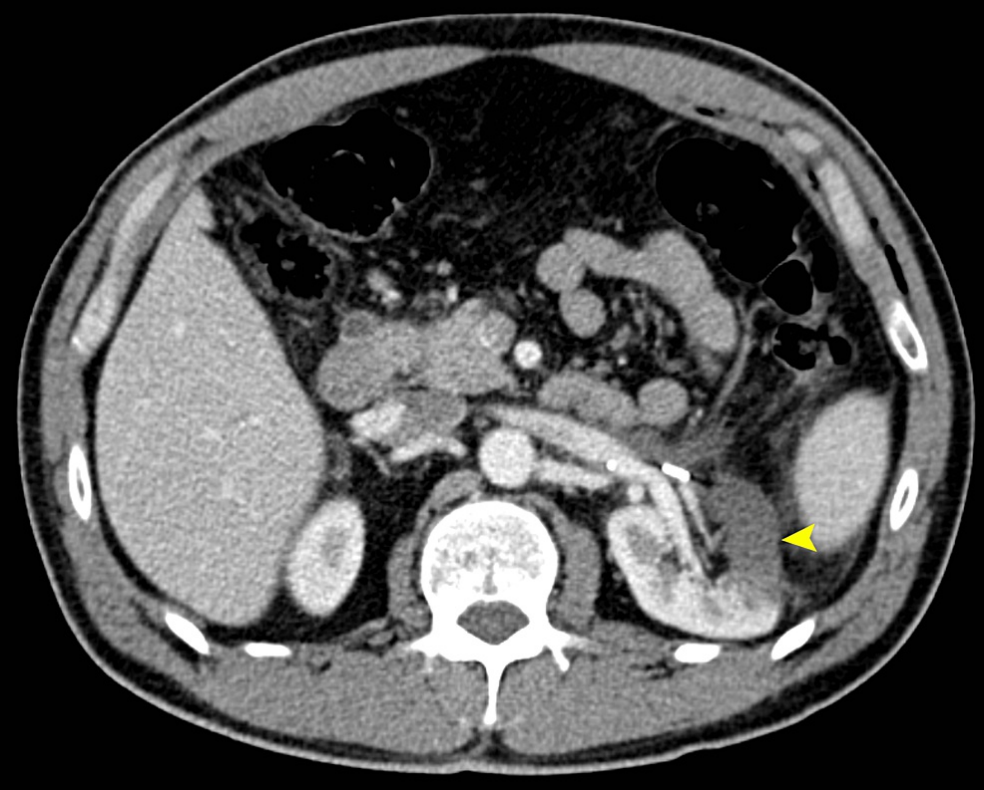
Abdominal CECT in corticomedullary phase, axial section over the renal hilum Observed is a wedge-shaped hypodensity over the anterior region of the left kidney due to segmental infarction. CECT: Contrast Enhanced Computed Tomography
